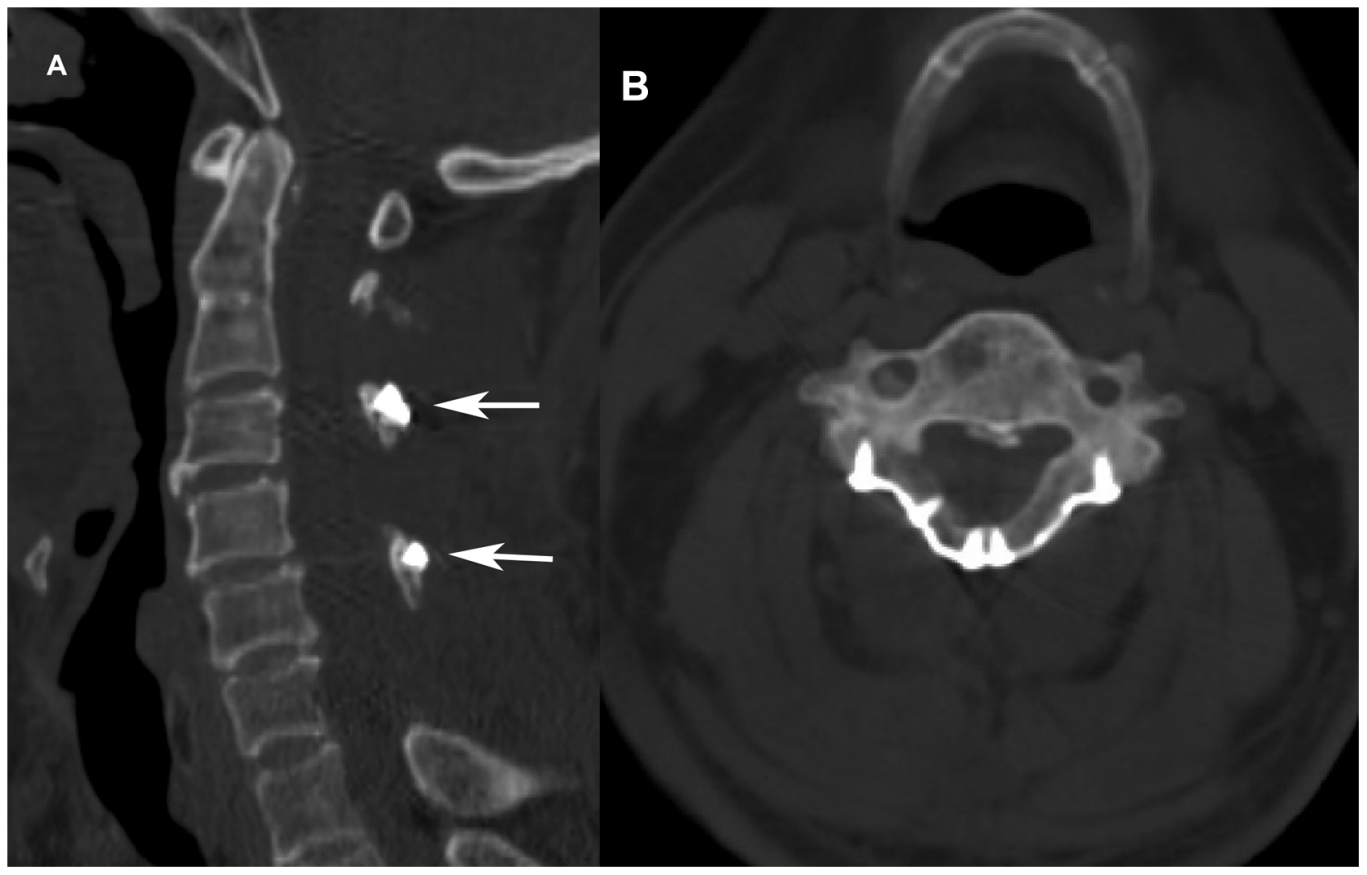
Postoperative CT scan of a patient 6 months after surgery. (A) Sagittal CT scan shows enlargement of the cervical spinal canal after posterior hybrid decompression. (B) Axial CT scan shows the reconstruction of a continuous and stable bony laminar arch. The hinge has completely healed with cortical bone on both its dorsal and ventral surfaces; the junction of bone block and host bone on the open side are bridged by cancellous bone.
